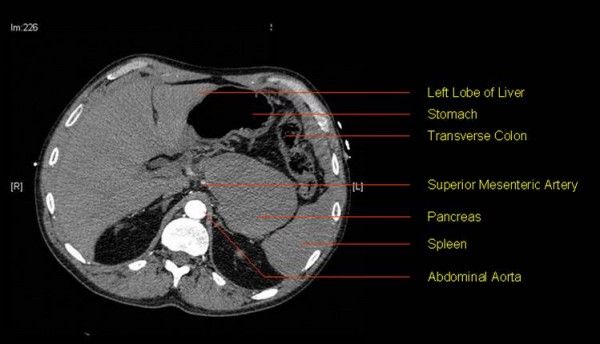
CT abdomen showing non-enhancing lesion in the tail of pancreas. Image in arterial phase taken before pancreas enhancement.
